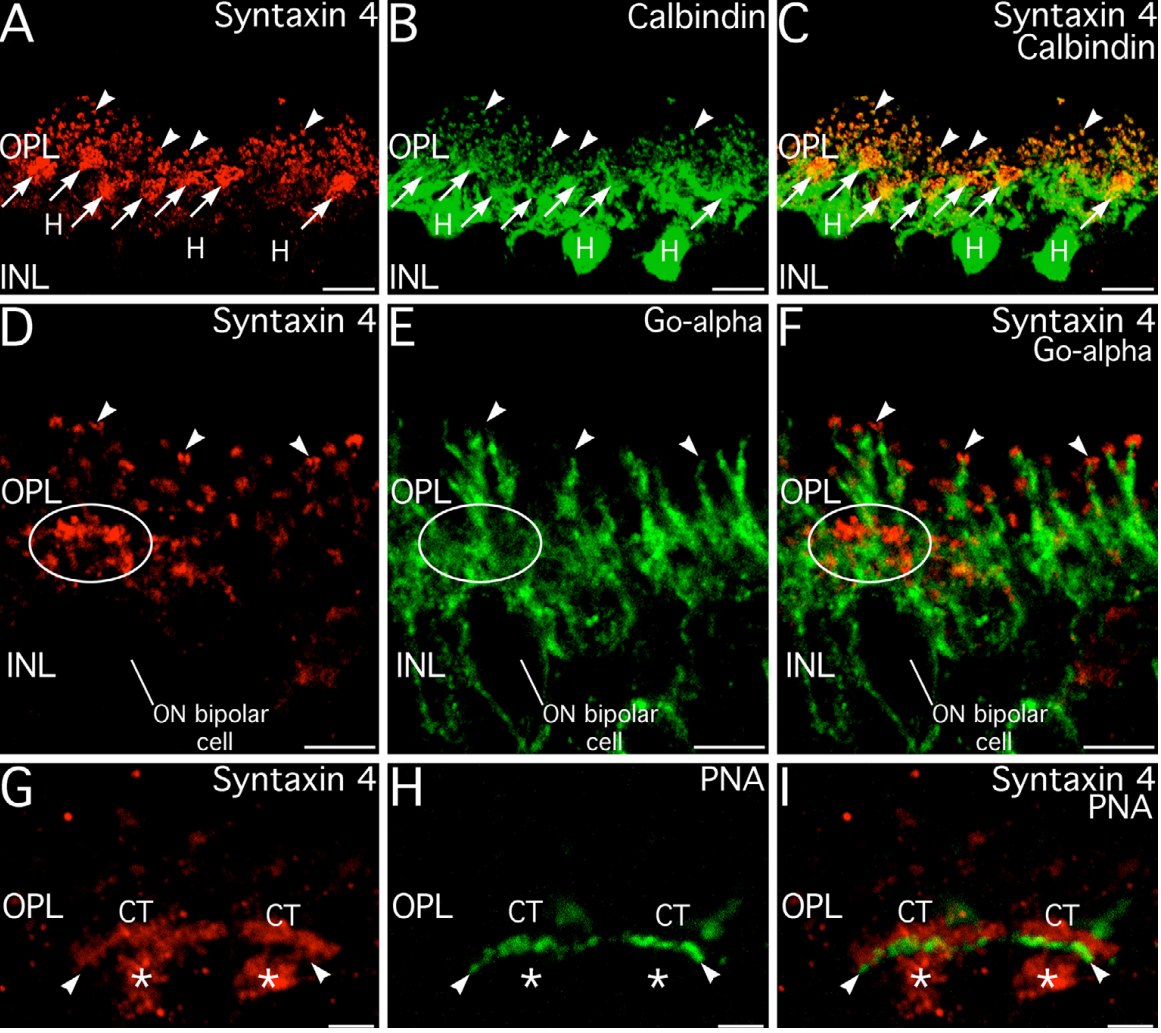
Syntaxin 4 is localized to the tips of horizontal cell processes at ribbon synapses, but not to dendrites of ON- or OFF-bipolar cells. A–C: Syntaxin 4 is present in the tips of horizontal cell processes in the ribbon synaptic complexes of rods and cones. Syntaxin 4 and calbindin, a horizontal cell marker, show double labeling in the individual puncta associated with rod terminals (arrowheads) and in the clusters of puncta associated with cone terminals (arrows) in the OPL, indicating that the syntaxin 4 is present at the tips of horizontal cell processes invading rod and cone terminals. Images shown from a projection of 37 optical sections with total thickness of 5.24 μm. D–F: Syntaxin 4 is not present in the dendrites of rod or ON-cone bipolar cells. Double labeling for syntaxin 4 and G_o_-alpha, a marker for rod and ON-cone bipolar cells, shows no colocalization. ON-bipolar cell dendrites reach up to the level of the syntaxin 4-positive puncta and terminate. In favorably oriented complexes in rod terminals (arrowheads), rod bipolar cell dendrites extending into the distal OPL terminate between the syntaxin 4-positive doublets corresponding to horizontal cell processes. No colocalization of syntaxin 4 and G_o_-alpha is present in ON-cone bipolar cell dendrites in cone terminals (ellipse). Image shown is a single optical section. G–I: Syntaxin 4 is not present at flat contacts between OFF-cone bipolar cells and cone terminals. Double labeling for syntaxin 4 and peanut agglutinin (PNA), a marker for flat contacts (arrowheads) between OFF-cone bipolar cells and cone terminals (CT) shows no colocalization. Syntaxin 4 labeling in processes directly proximal to the cone terminals is also visible (*). Images shown from a projection of 26 optical sections with total thickness of 6.71 μm. INL, inner nuclear layer. Scale bars = 10 μm for A–C; 5 μm for D–F; 2 μm for G–I.
